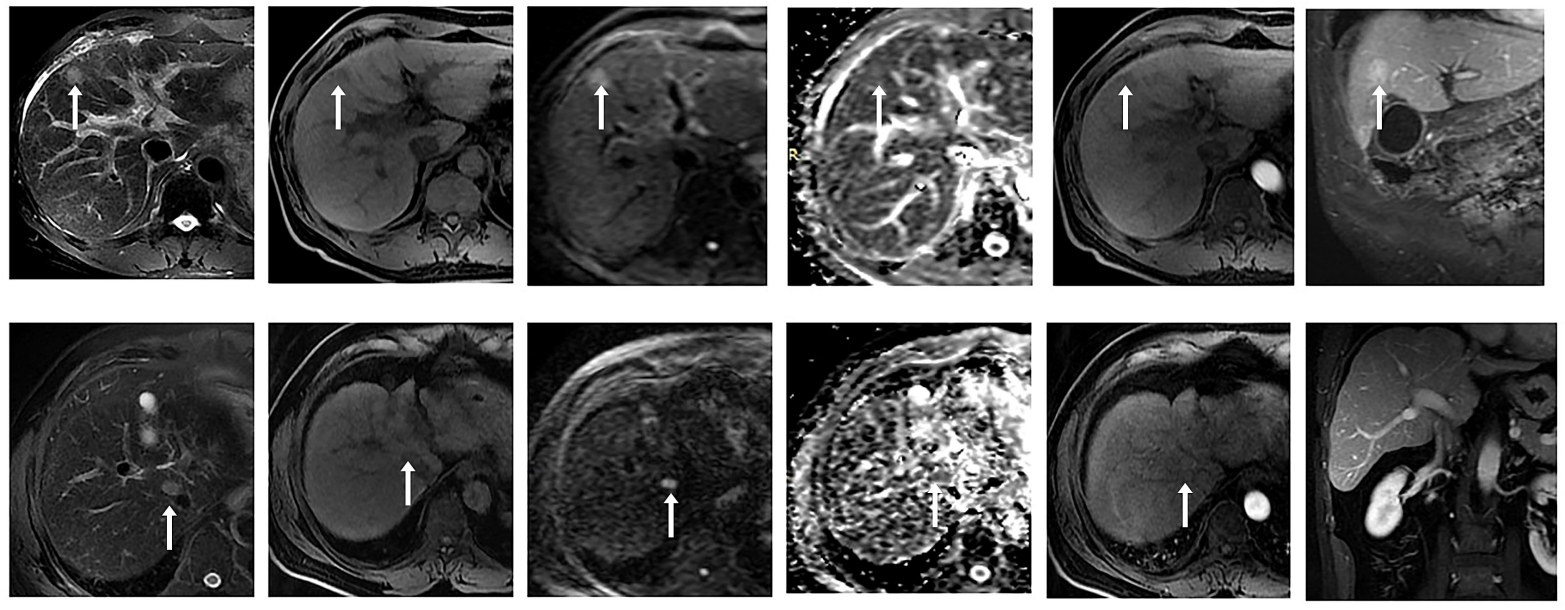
① Male, 53 years old, chronic hepatitis (B) A small nodule with a long diameter of about 1.8 cm in the left medial lobe of the liver showed high signal intensity on DWI (b value = 800 mm ²/s) and slightly high signal intensity on ADC, with a mean ADC value of about 1.409 × 10 ^-^ 3 mm ²/s. No significant enhancement was observed in the arterial phase after enhancement, and significant enhancement was observed in the portal venous and delayed phases. Postoperative pathology confirmed regenerative nodules (RN). ② A 58-year-old male patient presented with a history of chronic hepatitis B and cirrhosis. A small nodule with a long diameter of about 1.5 cm was observed at the junction of the right anterior lobe and caudate lobe of the liver, with low signal intensity on T1WI and slightly high signal intensity on T2WI. DWI (b value = 800 mm ²/s) and ADC maps showed limited diffusion, with an ADC mean value of about 0.983 × 10^-^ ³ mm ²/s. After enhancement, non-annular mild enhancement was observed in the arterial phase, isointensity was observed in the delayed phase, and no non-peripheral clearance or capsule-like enhancement was observed. Postoperative pathology confirmed hepatocellular carcinoma (HCC).
